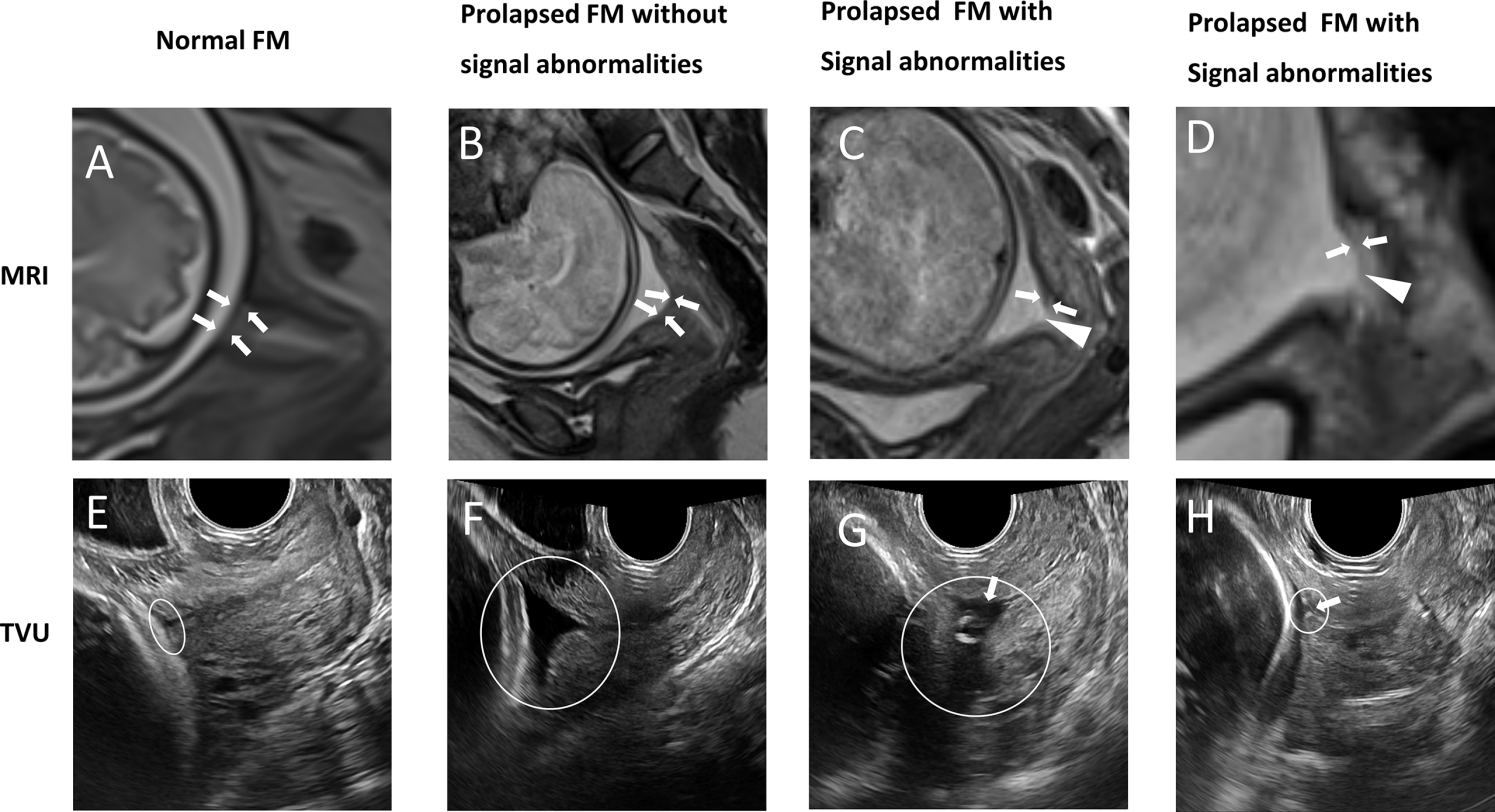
Patients underwent clinical MRI and transvaginal ultrasound (TVU) examinations on the same day. A vs. E (32wks); B vs. F (32wks); C vs. G (32wks); D vs. H (28wks). Panel (A–D) are MRI T2W images from the sagittal view. White arrows indicate the supra-cervical FM, and white triangles indicate fetal membrane defects. (A) Normal FM. (B) Prolapsed FM without signal abnormalities. (C) Prolapsed FM with signal abnormalities: partial defect of continuous FM signal. (D) Prolapsed FM with signal abnormalities: local thinning and increased signal intensity of continuous FM signal. The FM overlying the cervix is pointed by a white arrowhead. Panel (E–H) are TVU images. White rings indicate cervical internal os. (E) In the patient without prolapsed FM, the entire supra-cervical FM cannot be distinguished clearly due to the compression effect of the ultrasound probe. (F) In the patient with the prolapsed FM, the exact boundary between the supra-cervical FM and the underlying cervical tissue cannot be identified. (G, H) In patients with the prolapsed fetal membrane, the supra-cervical FM is partially visible. However, the signal abnormalities of the supra-cervical FM cannot be detected. White arrows point to the FM.
